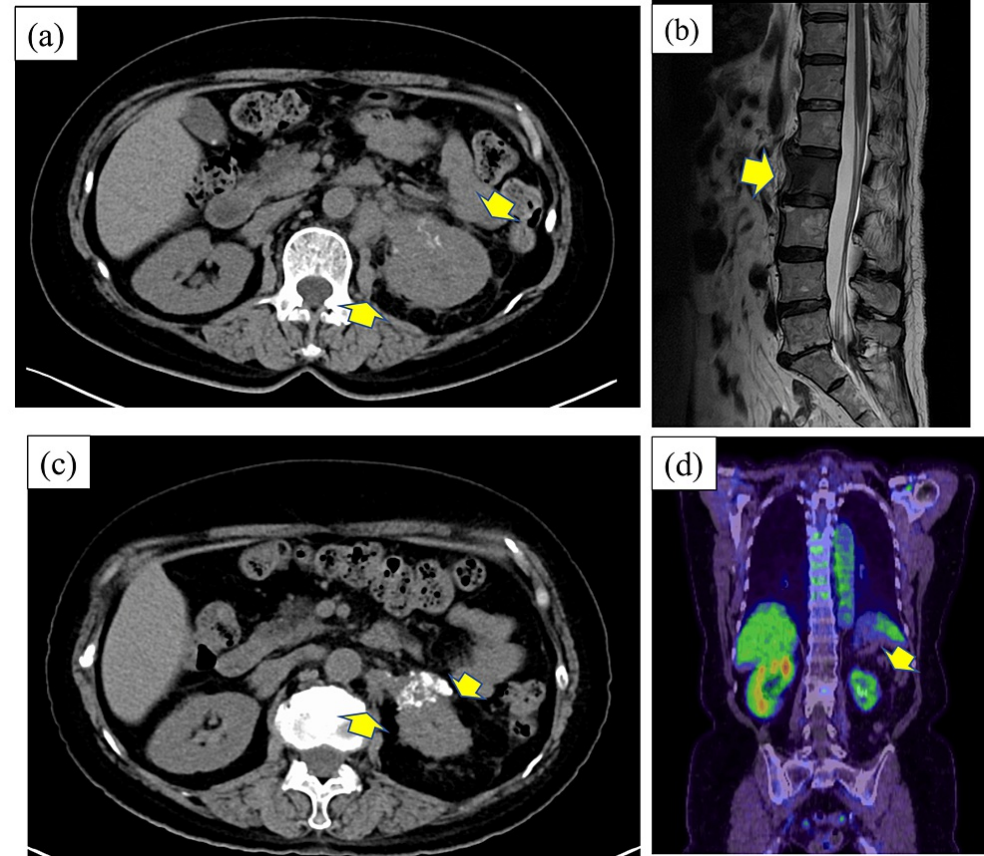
Computed tomography (CT), MRI, and PET-CT imaging. (a) Before treatment, CT scans revealed an oligemic mass in the left renal pelvis and an increased density of surrounding fatty tissue in the renal portal area, showing invasion of the tumor into the fat tissues. Periaortic lymph node metastasis was also noted (cT3N2M0). (b) MRI showed spinal metastasis of the clear cell variant into L2. (c) The tumor volume was significantly decreased after enfortumab vedotin (EV) second-line treatment. (d) PET-CT showed that FDG uptake was weak in the left kidney. Because the uptake was significantly low, this indicated that the tumor viability was suppressed by the treatments. The FDG accumulation in the surrounding lymph nodes was also extremely low. FDG: fluorodeoxyglucose.
